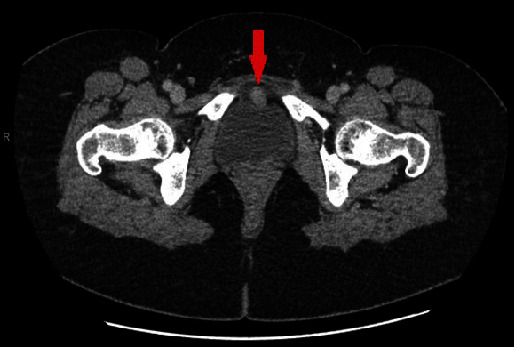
Contrast-enhanced CT scan with 5 mm focus enhancement on the right anterolateral wall of the urinary bladder.
